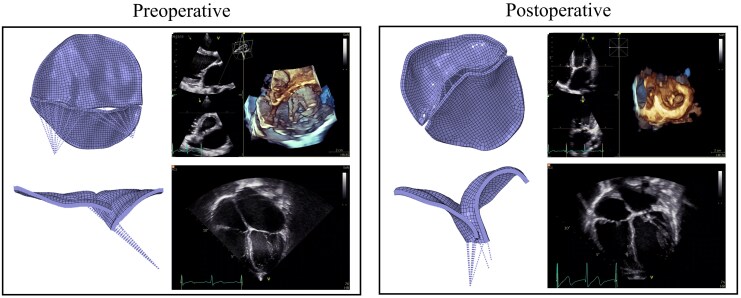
The valve segmentation. The pre- and postoperative segmentations for Patient 2 with the corresponding 3D echocardiography, at end-systole.
